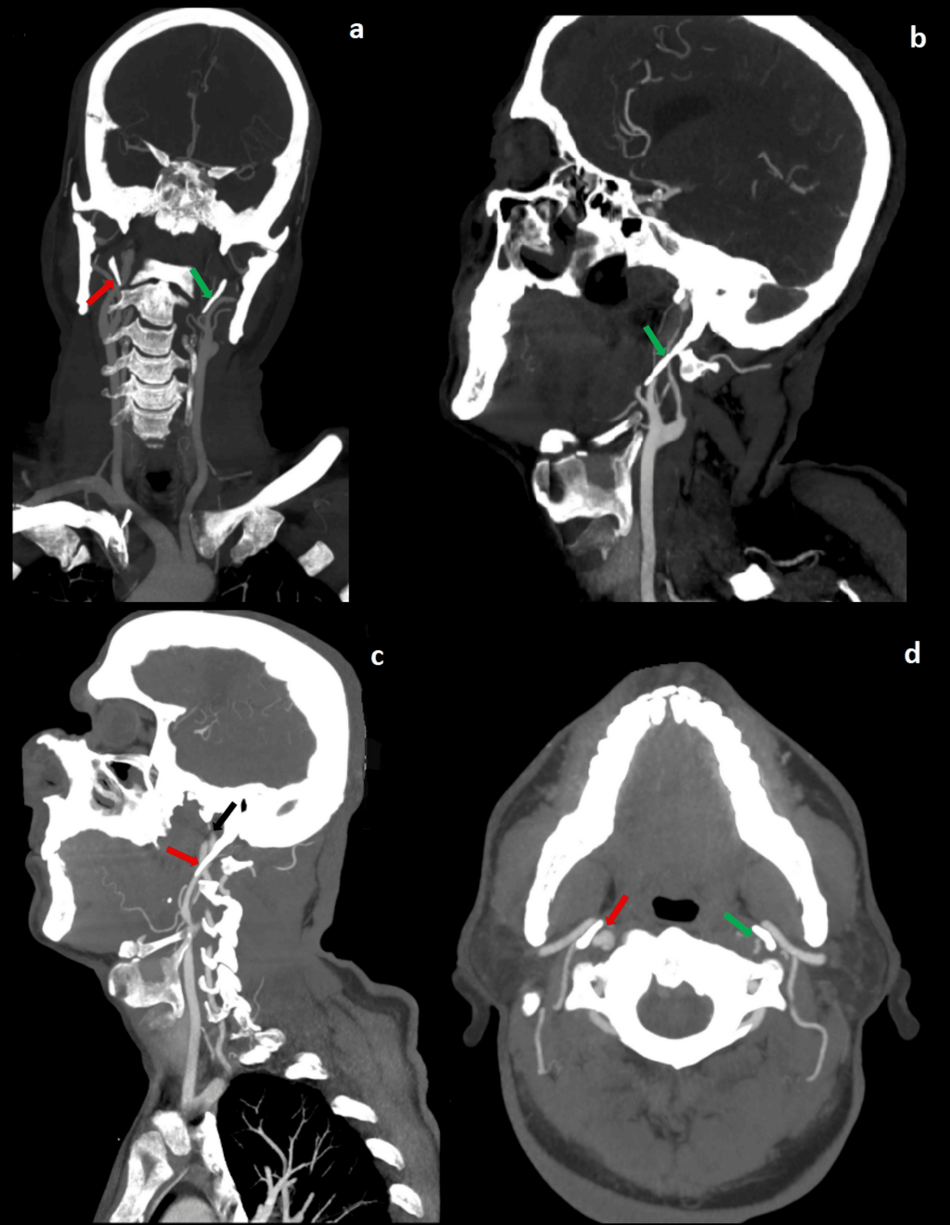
Case 6 frontal (a), sagittal (b c), and axial CT (d) images showing bilateral carotid artery dissection caused by conflict with elongated styloid processes (42.3 mm on the left and 41.3 mm on the right). Red arrows indicate the right carotid artery, and green arrows indicate the left carotid artery. The conflict results in a pseudoaneurysm of the right ICA (black arrow) CT: computed tomography, ICA: internal carotid artery
